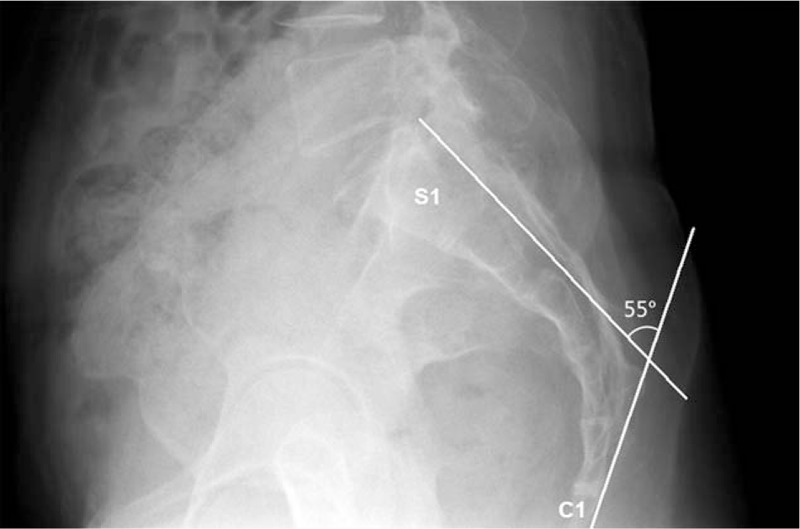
Measurements of the sacral angle using plain radiography. The sacral angle was defined that the angle between the extension line from the posterior body of the first sacral segment (S1) and the extension line from the posterior body of the first coccygeal segment (C1) in a lateral view.
